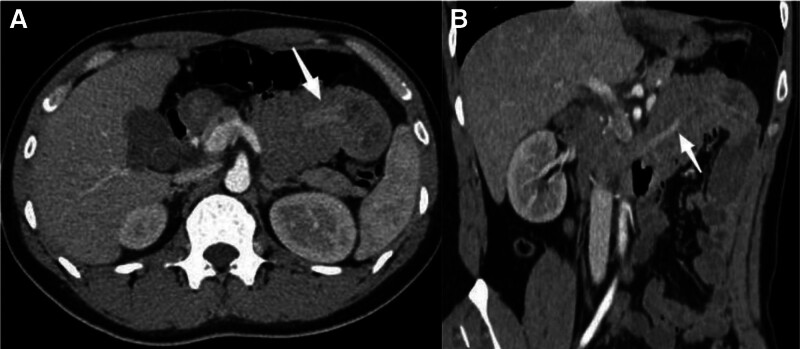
Arterial-phase CT image showing long strip-shaped abnormally enhanced shadows in the duodenum, accompanied by obstruction and dilation of the horizontal and jejunal segments of the duodenum. CT = computed tomography.
